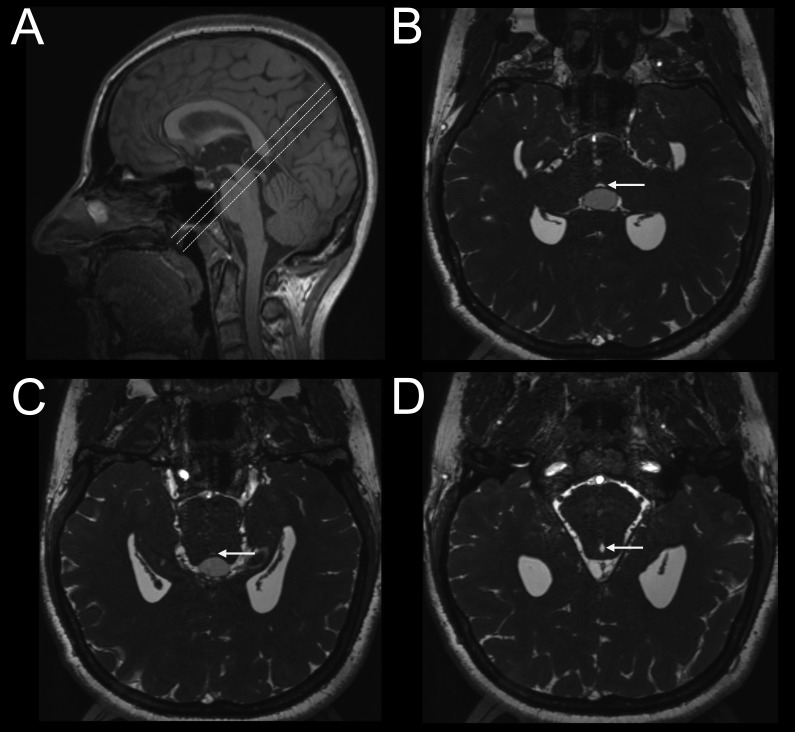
(A) Sagittal T1 slice with three dotted lines representing the planes used for magnetic resonance imaging (MRI) analysis of the aqueduct of Sylvius. (B) Constructive interference of steady state (CISS) T2 sequence reformatted to the plane of the most cranial dotted line showed compression of quadrigeminal plate with pronounced stenosis of aqueduct of Sylvius. (C) CISS T2 sequence reformatted to the plane of the middle dotted line showed near-obstruction stenosis of the aqueduct of Sylvius. (D) CISS T2 sequence reformatted to the plane of the most caudal dotted line showed a preserved lumen of the aqueduct of Sylvius in the caudal part of canal.
